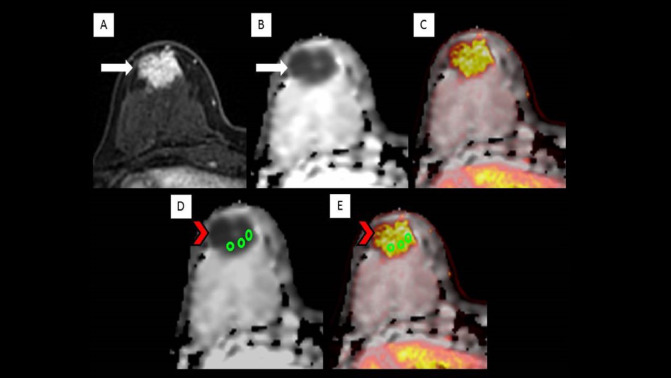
ROI placement for apparent diffusion coefficient (ADC) measurement in invasive ductal carcinoma (IDC). (A) Early-phase dynamic contrast-enhanced MRI (DCE-MRI) demonstrating tumour enhancement. (B) Corresponding ADC map showing restricted diffusion within the lesion (arrow). (C) Fusion of subtraction DCE-MRI and ADC map to guide ROI placement. (D) Single manually drawn region of interest (ROI) placed on the ADC map, avoiding necrotic or cystic components. (E) Fusion images confirming ROI placement within viable tumour tissue. A low-intensity region on the ADC map (arrowhead) demonstrates reduced enhancement on DCE-MRI and was excluded from analysis, consistent with necrotic change. ADC = apparent diffusion coefficient; DCE-MRI = dynamic contrast-enhanced magnetic resonance imaging.
